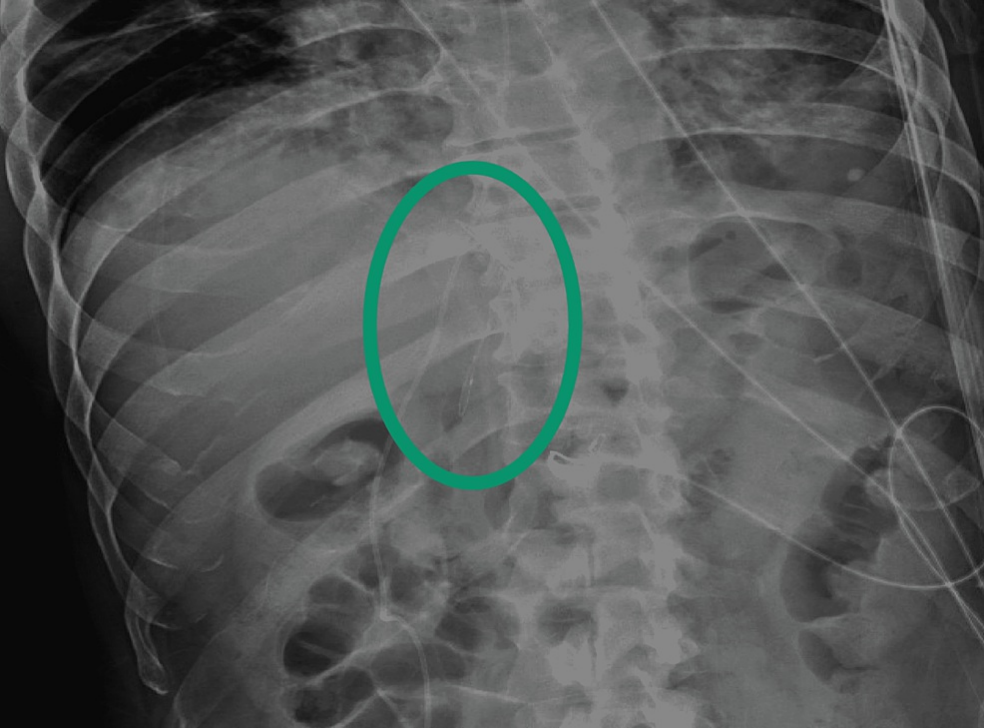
Abdominal X-ray showing the IVC filter IVC: Inferior Vena Cava
